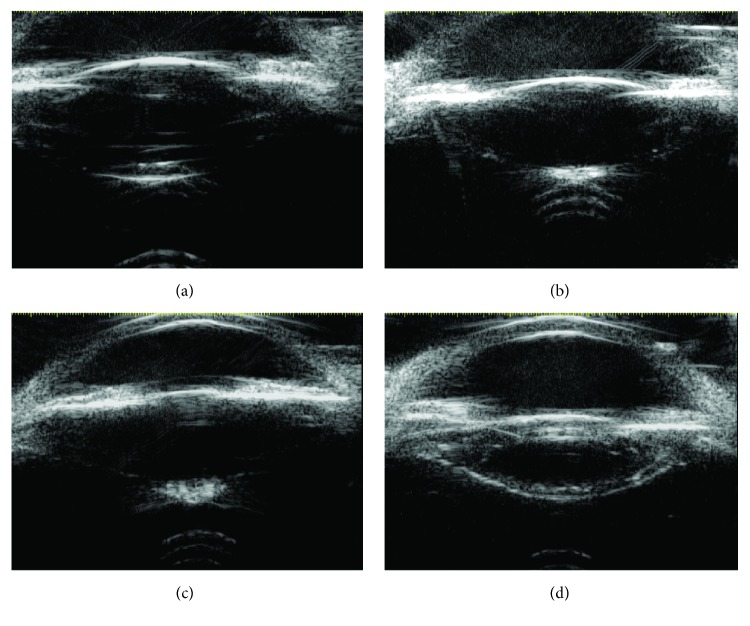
Images of the posterior capsule (PC) under 25 MHz B-scan ultrasonography (MHzB). (a) Normal continuous PC curve (CPCC) with minimal reflectivity between the PC and posterior polar cataract (PPC) in a clinically diagnosed case of PPC. (b) Normal CPCC with high reflectivity between the PC and PPC in a clinically diagnosed case of PPC. (c) PC dehiscence with reflectivity protruding into the vitreous cavity in a clinically diagnosed case of PPC. (d) Posterior subcapsular cataract with an intact PC.
